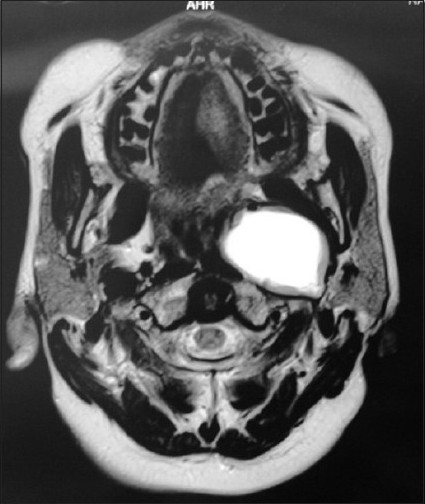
MRI axial view showing a neurofibroma in the left infra temporal fossa
